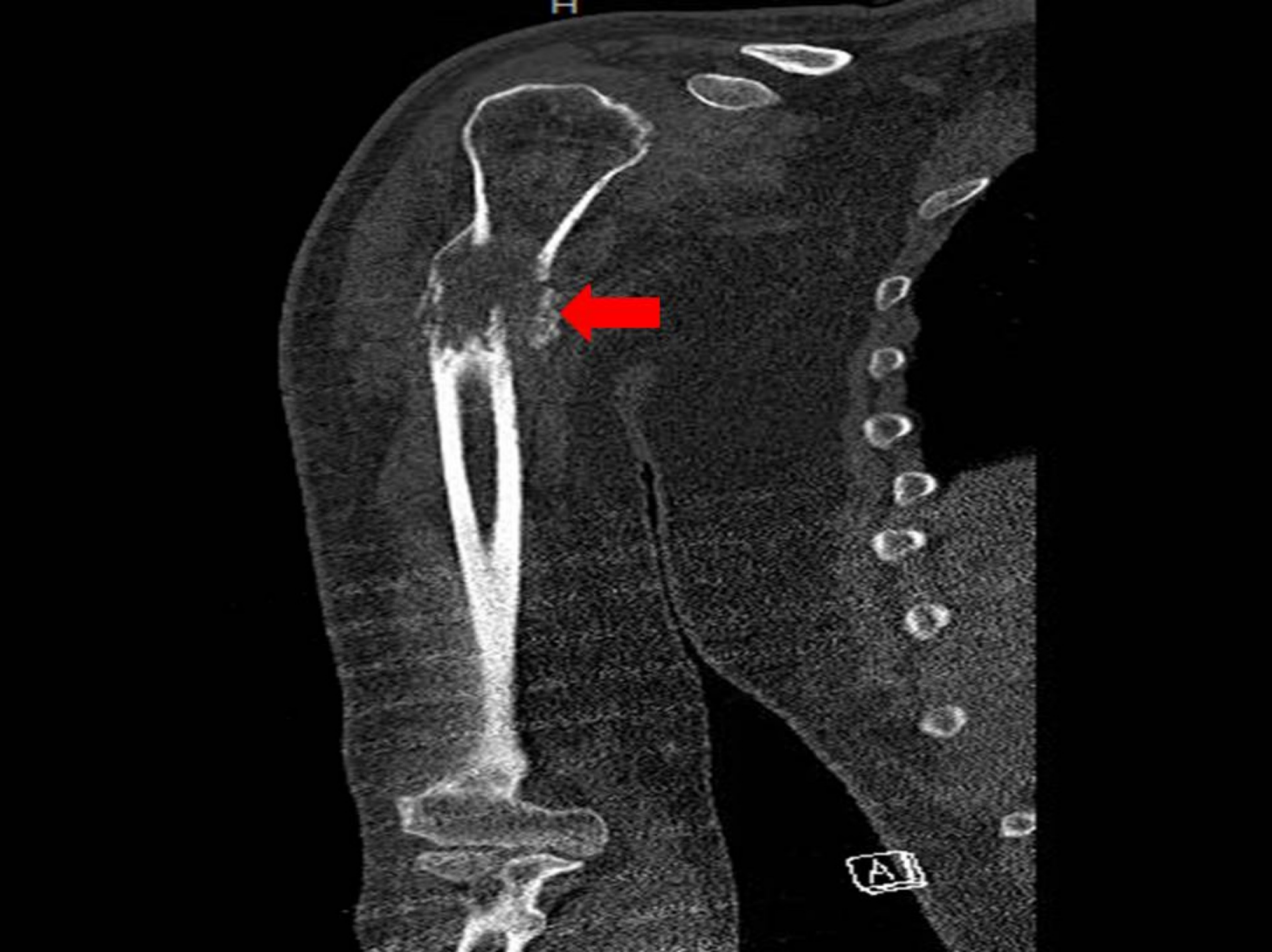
Computed tomography scan of the right arm showing a mass lesion (red arrow) in the proximal diaphysis of the humerus, invading the intramedullary canal and extending beyond the boundaries of the humerus.
